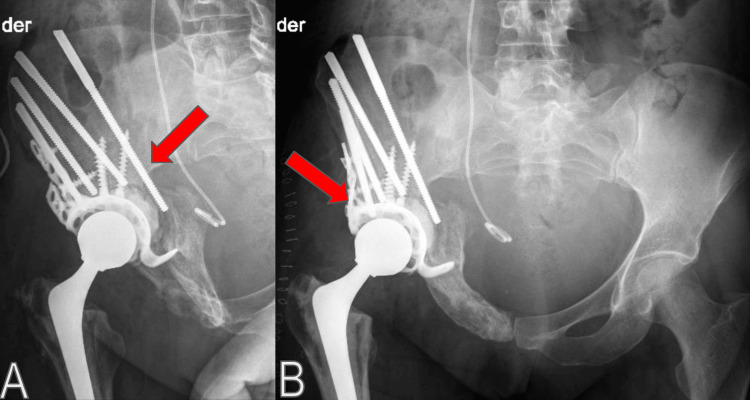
Post-operative radiographs Alar (A) and anteroposterior (B) views showing the final reconstruction, with the distribution of the four hydroxyapatite-coated Schanz pins and acetabular cage, and reinforcement of the weight-bearing area with screws and cement (red arrows).
